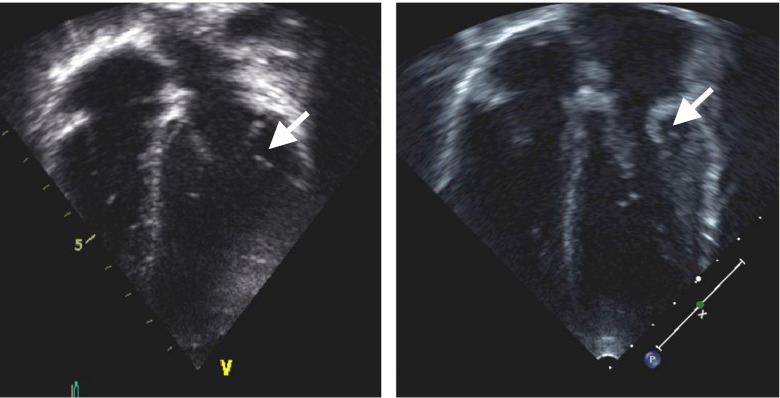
Echocardiographic images showing a normal mitral valve (*left*) and a thickened mitral valve in a patient with mucopolysaccharidosis (MPS) VI (*right*)
